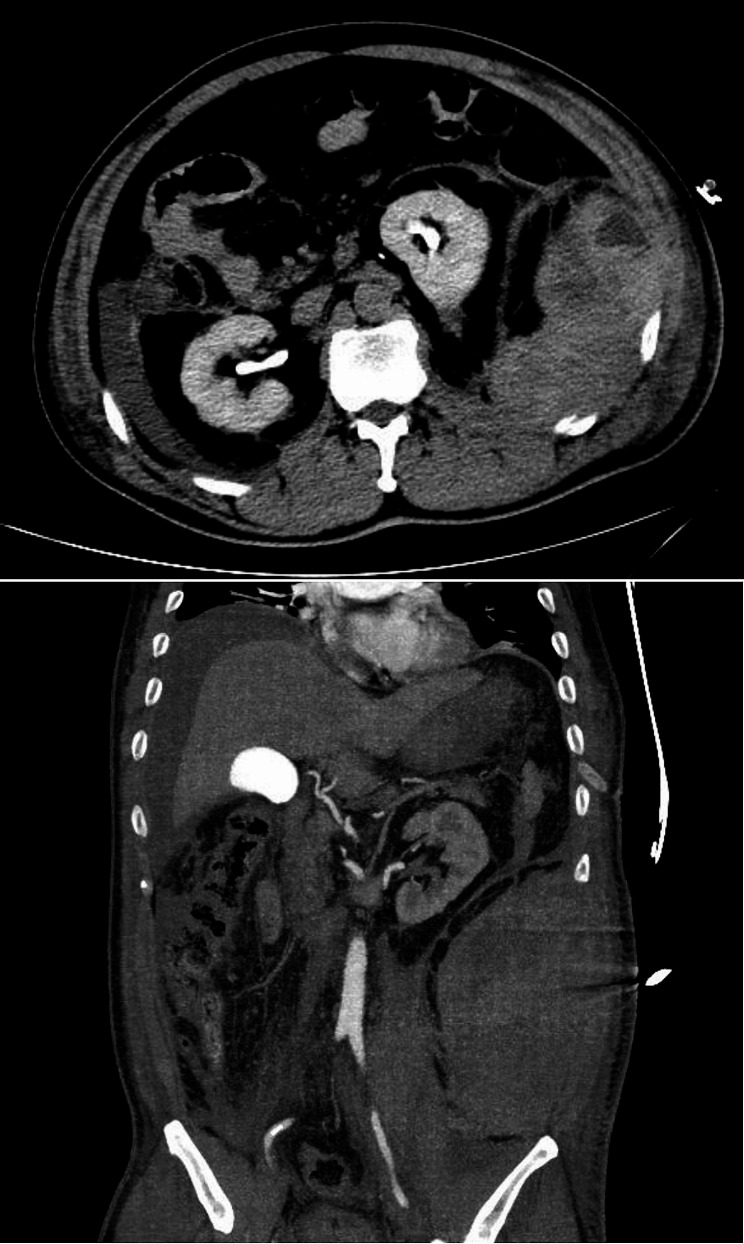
CT scan reveals the fractured end of the rib and retroperitoneal hematoma
